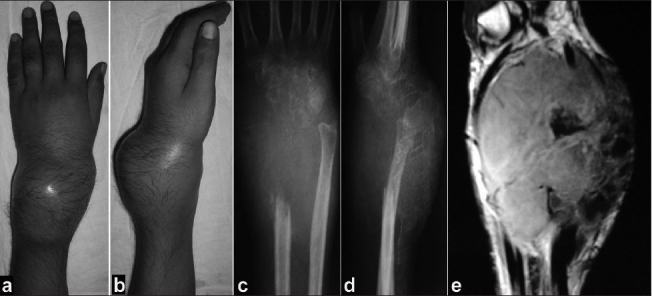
Clinical photograph dorsal (a) and side (b) views, with giant cell tumour of the lower end of radius. Anteroposterior (c) and lateral (d) radiograph (e) saggital MRI showing extensive bony destuction campanacci grade III giant cell tumour
